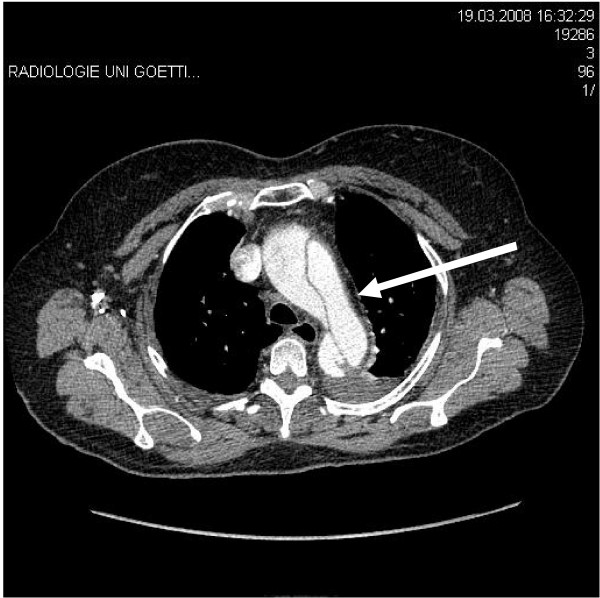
CT-scan showing type A dissection with a dissection flap.
